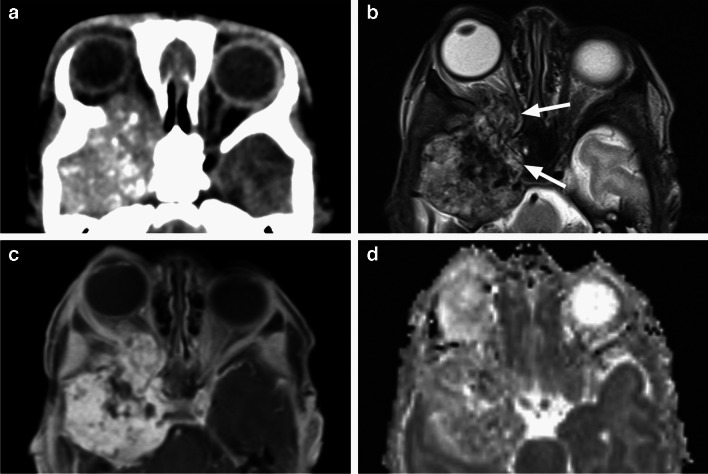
Congenital hemangioma. Axial CT image (a) in a neonate with prominent proptosis shows a heterogeneous right intraorbital and intracranial mass containing calcifications. Follow-up MRI scan with axial T2-weighted image at a similar level (b) reveals flow voids (arrows) within the heterogenous mass (arrows). Corresponding postcontrast T1-weighted image (c) shows intense enhancement of the lesion, while ADC map (d) demonstrates increased diffusivity (hyperintensity compared to the brain)
